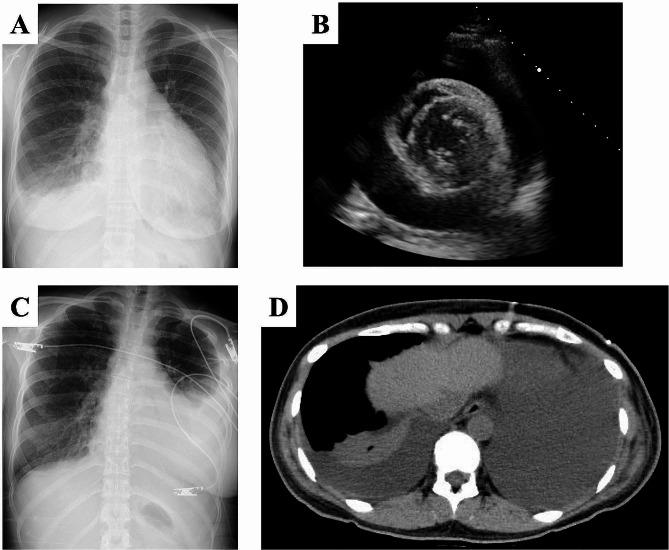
Imaging findings of cardiac tamponade and acute pleuritis after thymectomy. Chest radiograph shows obvious cardiomegaly with a 62% cardio-thoracic ratio (A), and an echocardiographic parasternal short-axis view shows massive pericardial effusion (B). After pericardial drainage, chest radiograph shows pleural effusion in the left thoracic cavity (C), and chest computed tomography shows bilateral pleural effusions, especially on the left, with massive pleural effusion and the heart shifted to the right. Pericardial effusion is hardly observed (D)
